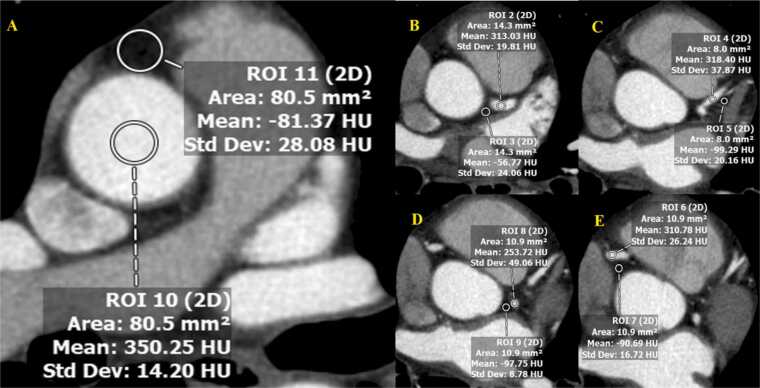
Axial coronary computed tomography scans demonstrate the five anatomic locations on which the signal-to-noise ratio and contrast-to-noise ratio were measured. A. mid-ascending aorta, B. Left main coronary artery, C. Left anterior descending artery, D. Left circumflex artery, and E. Right coronary artery.
